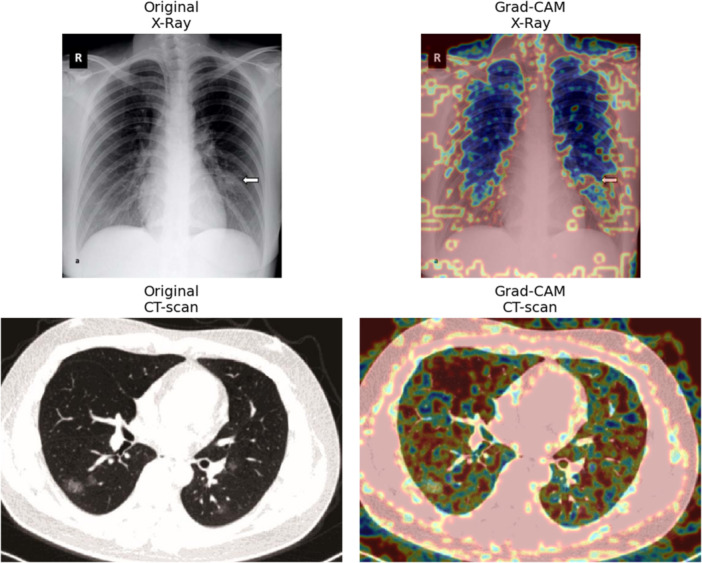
Grad‐CAM visualizations for X‐ray and CT‐scan images.
